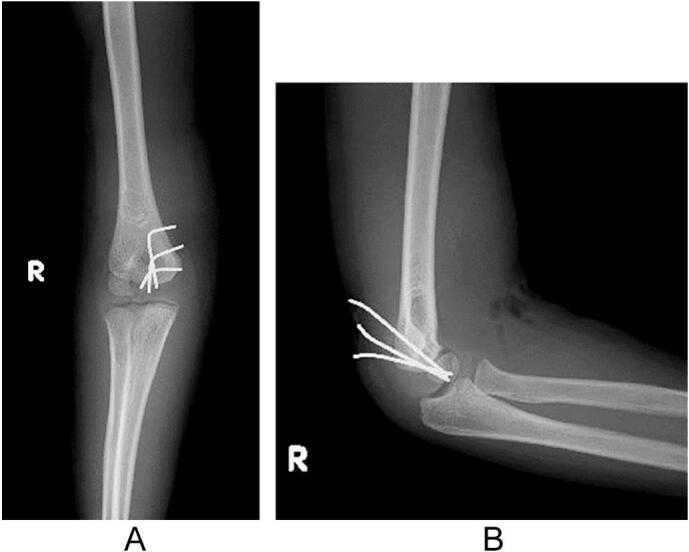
Postoperative plain X-ray of the right elbow. (a) Anteroposterior view. (b) Lateral view.
